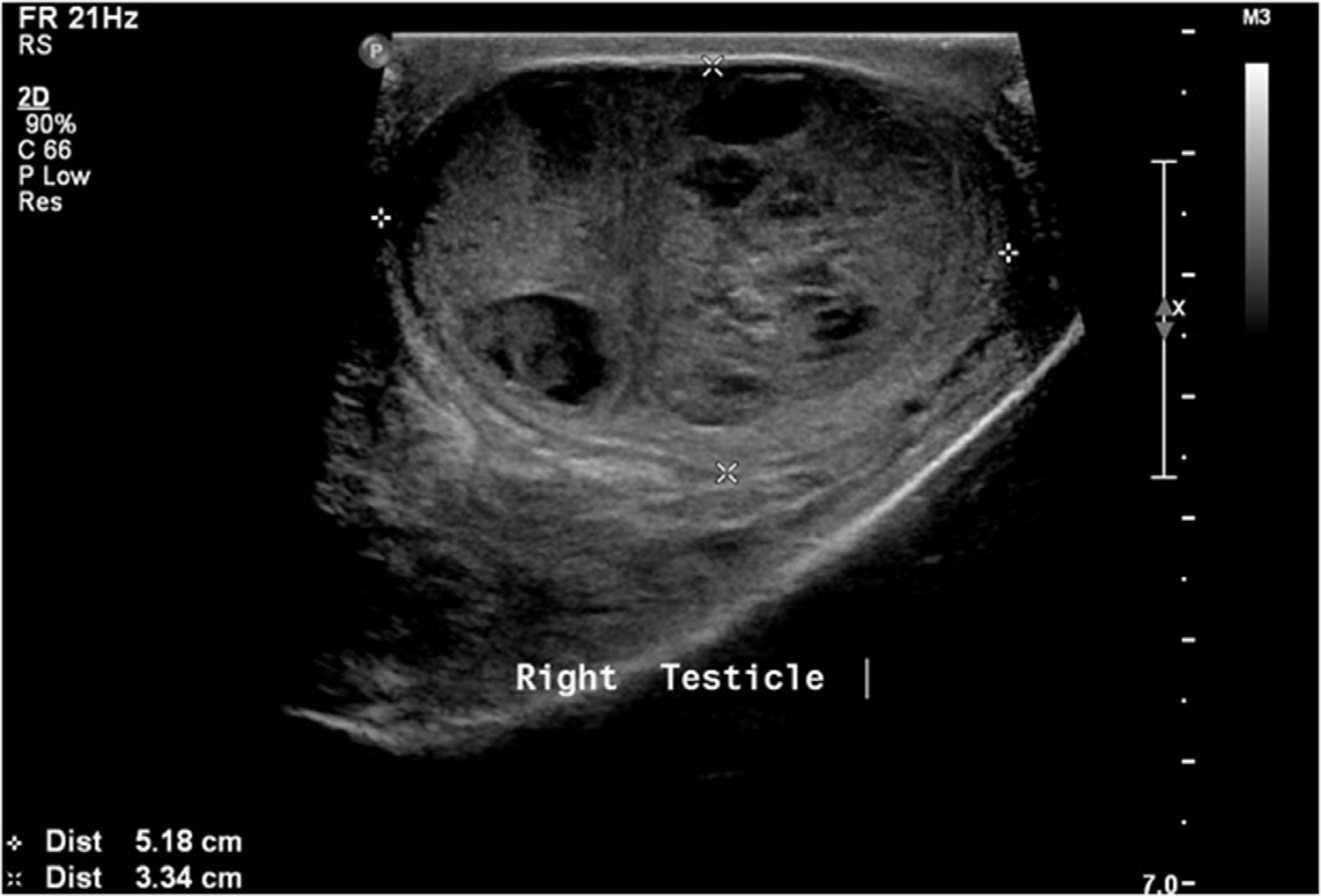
Grayscale ultrasound of the right testis in long axis showing the parenchymal heterogeneous mass lesion with areas of cystic changes/necrosis. Note the peripheral normal testicular tissue.
